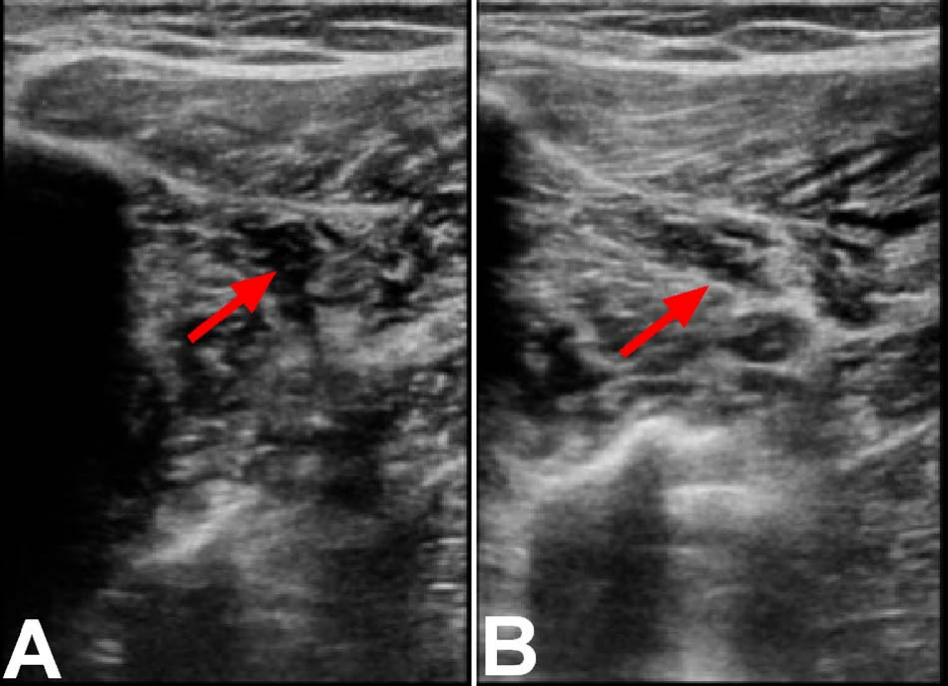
Ultrasound of the right lower extremity within the anterior peroneal vein prior to compression (A) and with compression (B). Incomplete compression of the vein is suggestive of DVT as indicated by the red arrows.
